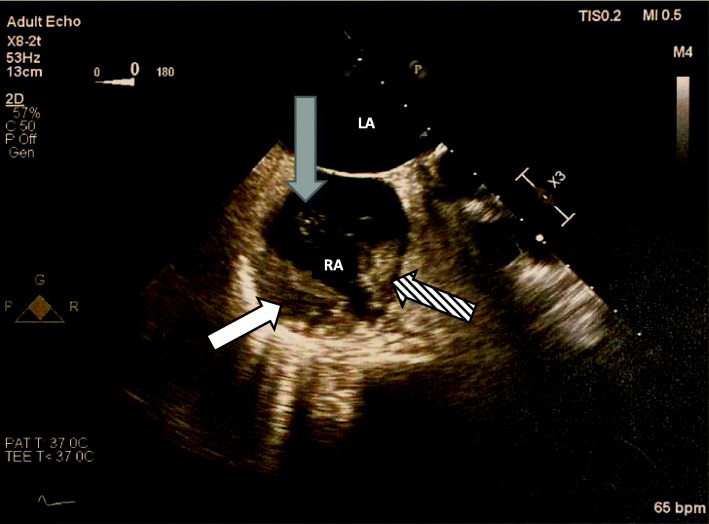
Transesophageal Echocardiogram (TEE). White arrow demonstrating immobile right atrial thrombus. Striped arrow demonstrates second mobile thrombus. Grey arrow demonstrates thrombin strands originating from mobile thrombus
